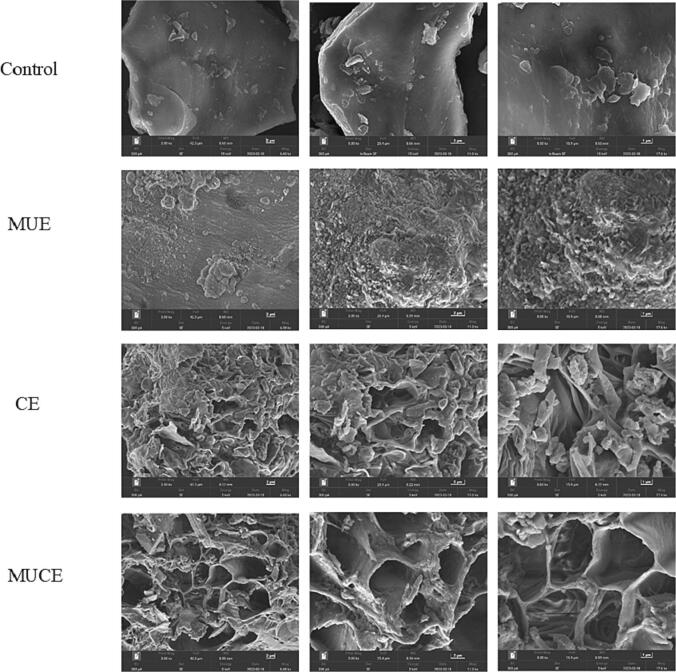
SEM images of the mulberry leaf by different extraction methods. The magnification was 3000×, 5000×, and 8000×, respectively. (Control: traditional extraction; MUE: multi-frequency ultrasound extraction; CE: cellulase extraction; MUCE: multi-frequency ultrasound-assisted cellulase extraction).
